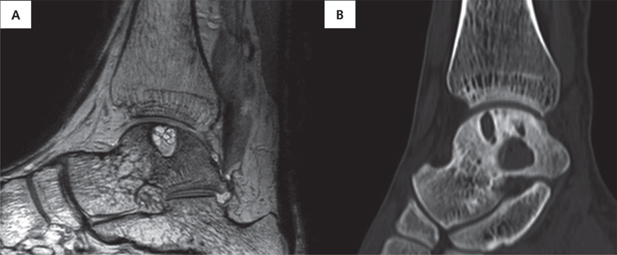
A 59-year-old man with OCL. (A) Initial MRI and (B) CT at 4-year follow-up. Although we recommended surgery to the patient at the first visit, the patient refused the operation because the symptoms were tolerable. The lesion size was increased on the CT at 4-year follow-up. However, the patient did not have worse symptoms and still did not want surgery.
